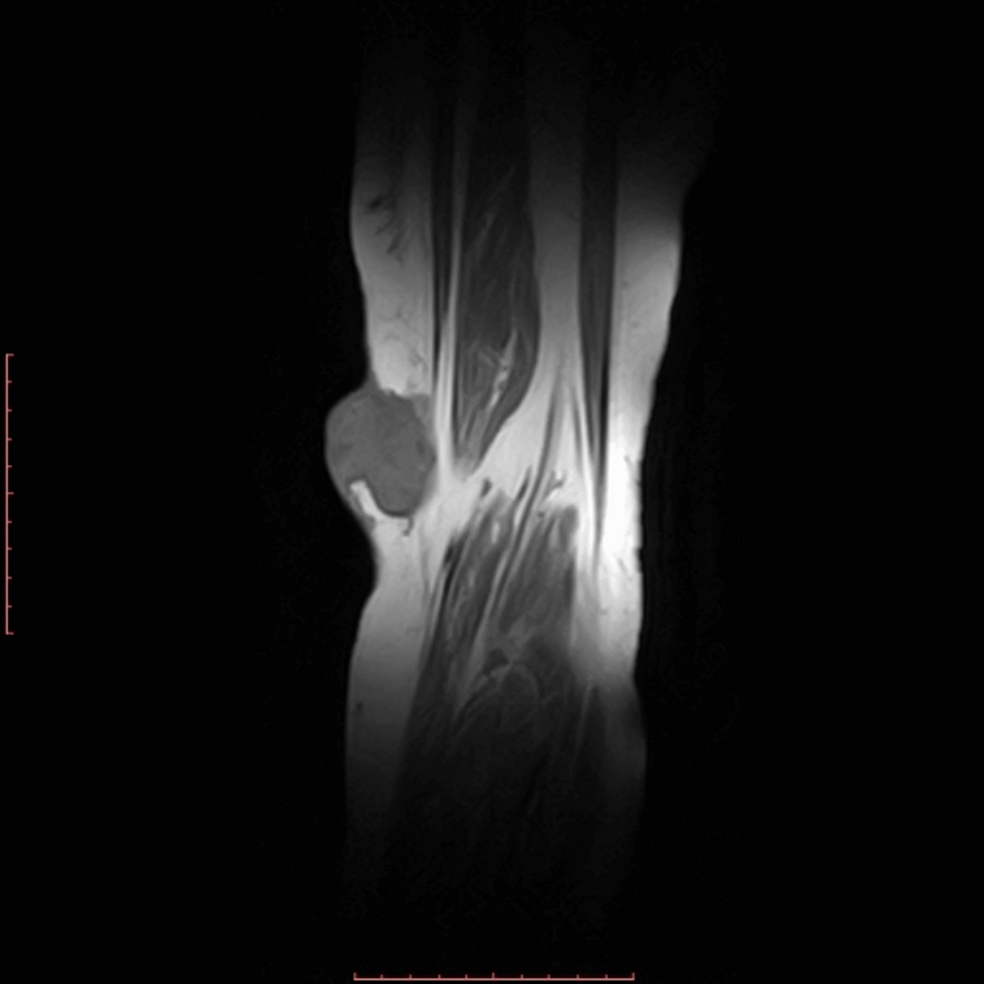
Magnetic resonance imaging: T1 coronal slice showing a well-circumscribed mass, both solid and cystic components of the lesion in the subcutaneous plane of the medial popliteal fossa can be seen. No invasion of soft tissue, joint space, or bone.
